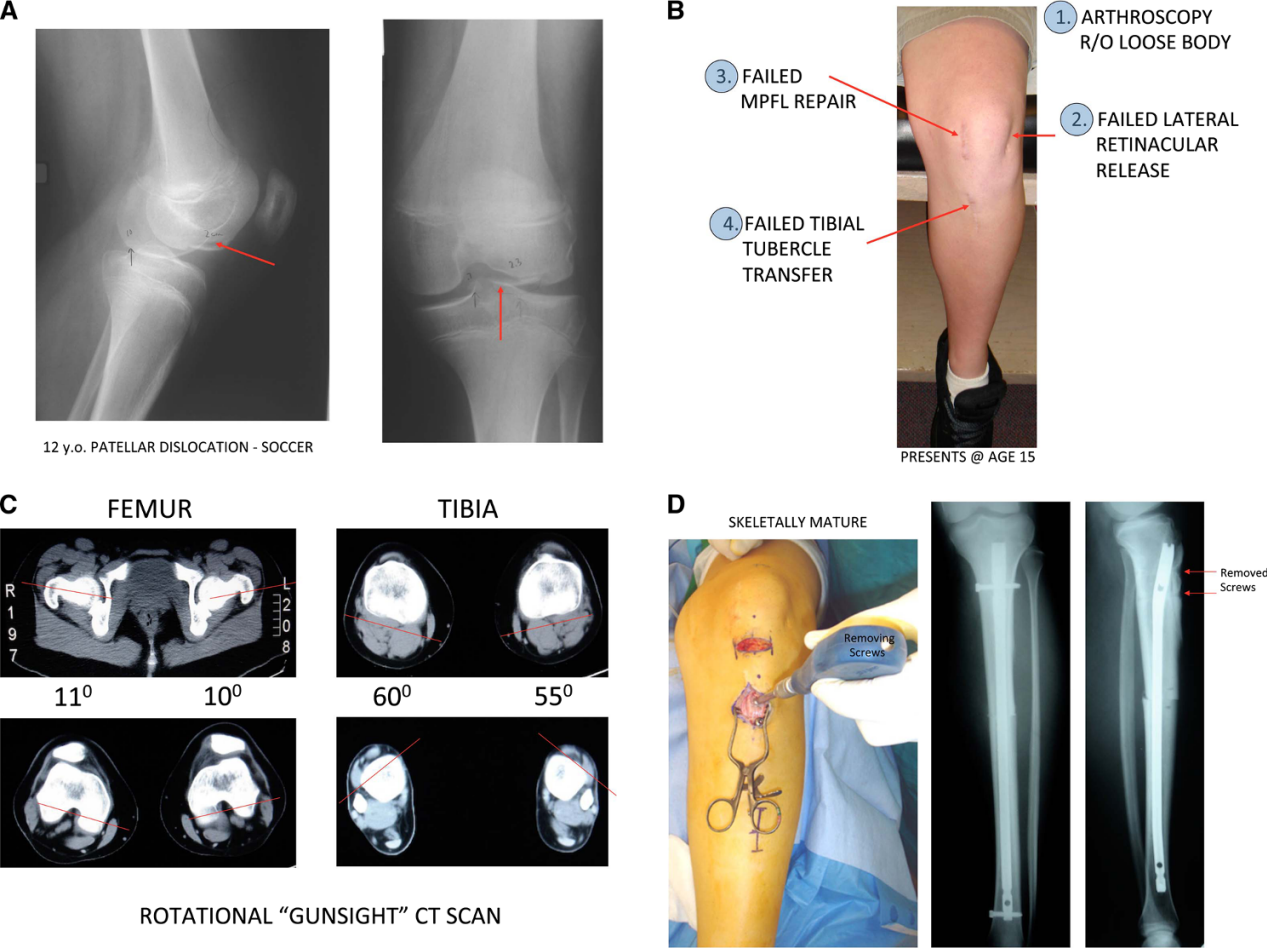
a A 12-year-old girl was “tackled” playing soccer and suffered a traumatic dislocation of her *left* patella. The *arrow* depicts the osteochondral fracture. b Three years (and four knee operations) later, she continues to have *left* patellar instability. She is now developing knee symptoms on the *right*. c Her torsional profile demonstrated 30° of excessive outward tibial torsion bilaterally. Her “gunsight” CT scan corroborates the excessive bilateral tibial outward torsion, in the presence of normal femoral version. d At the time of her 30° rotational tibial osteotomy, the tubercle screws were removed, leaving the transferred tubercle in situ. Two months later, she underwent a rotational osteotomy of her *right* tibia. At latest follow-up, she has stable patellae and is asymptomatic
